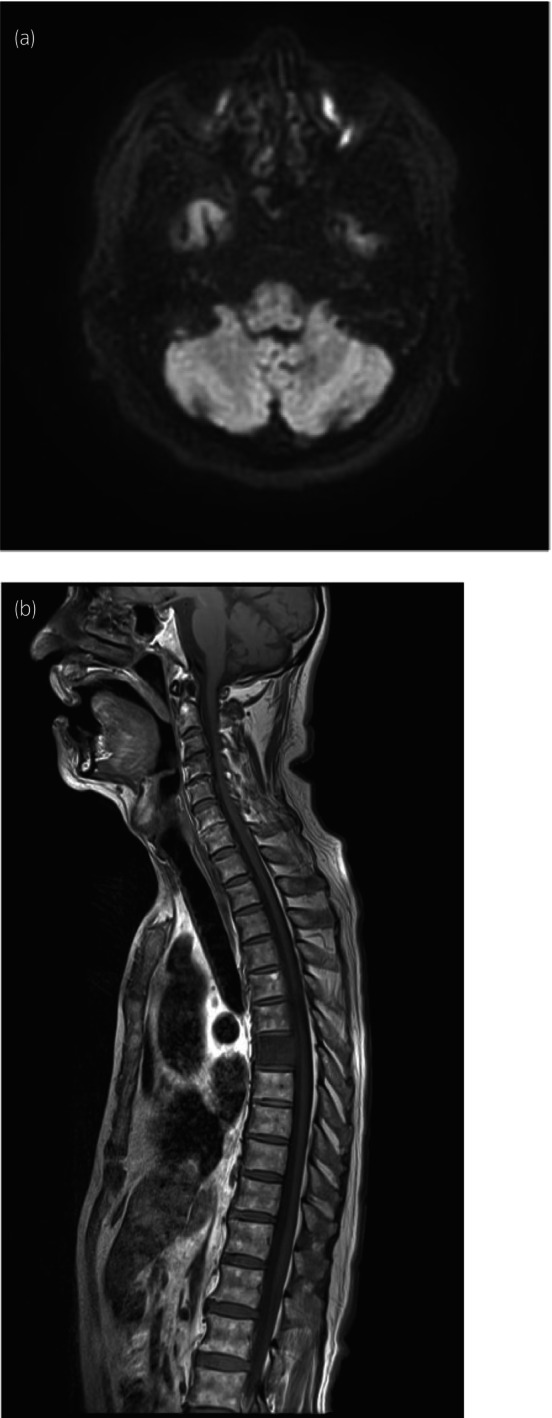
MRI upon close inspection. (a) MRI of the head showing no obvious abnormal findings. (b) MRI of the spinal cord showing no obvious abnormal findings.
